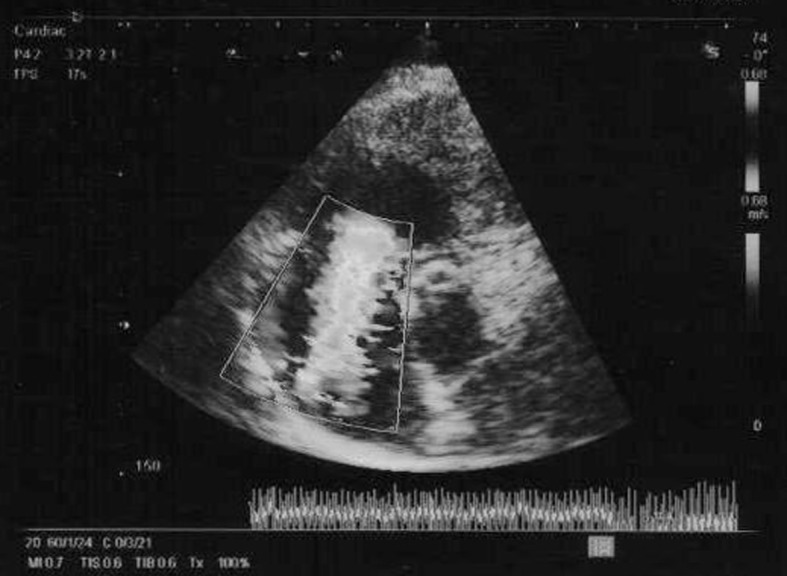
Two dimensional echocardiography showed a hyperactive, dilated right ventricle with rigid immobile tricuspid valve leaflets and moderately severe tricuspid regurgitation, dilated right atrium and normal aortic and pulmonary valves.
